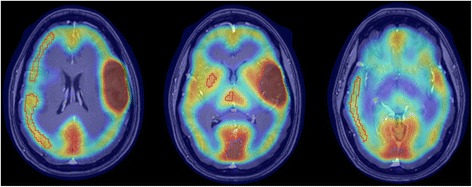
AMT-PET/T1-Gad MRI fusion images of a patient (#10) with left frontal WHO grade 2 glioma. Contralateral frontal, parietal, and temporal cortical, as well as thalamic and striatal regions of interest used for analysis, are outlined in *red*
